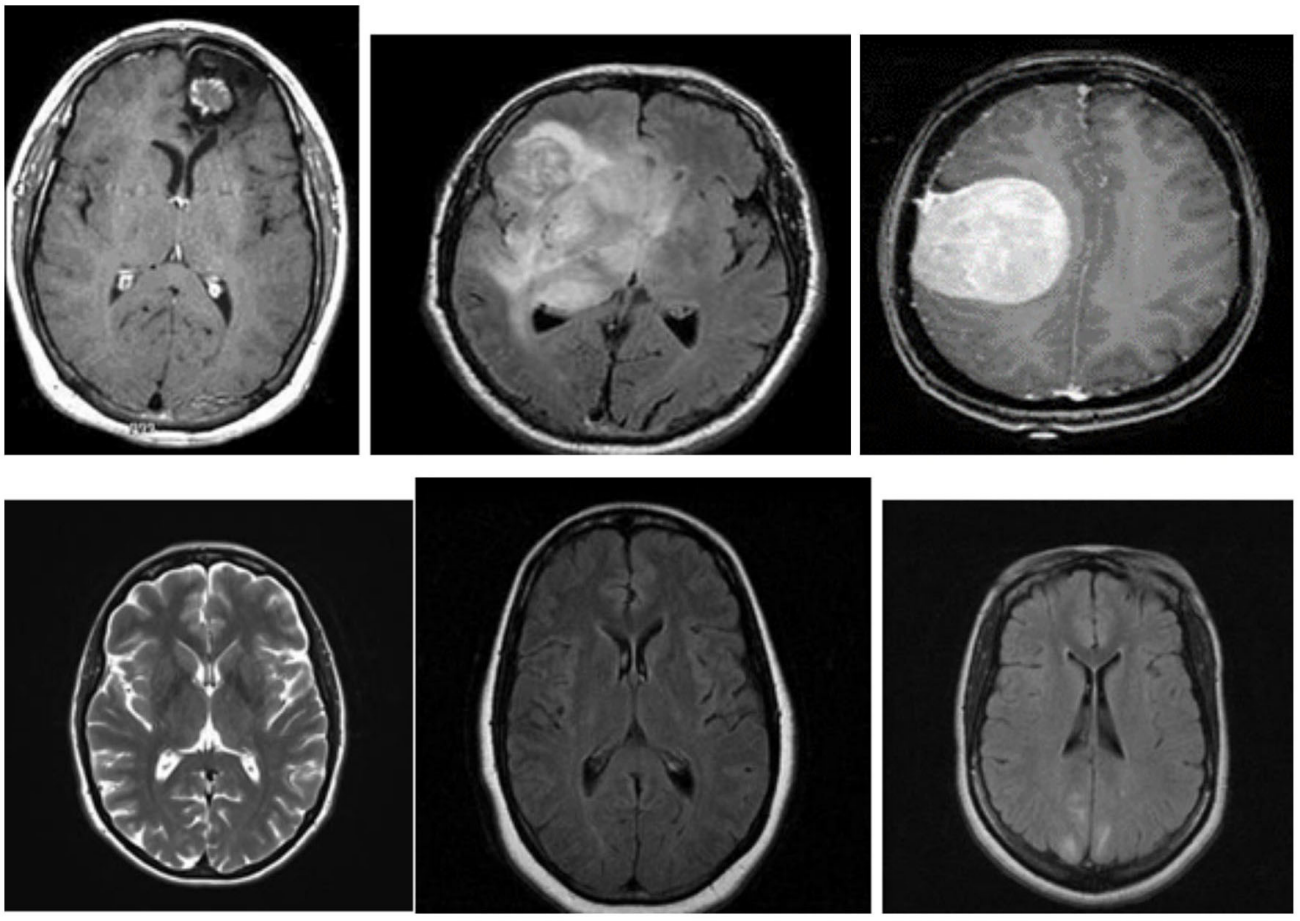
MR images with and without tumor from the dataset.
